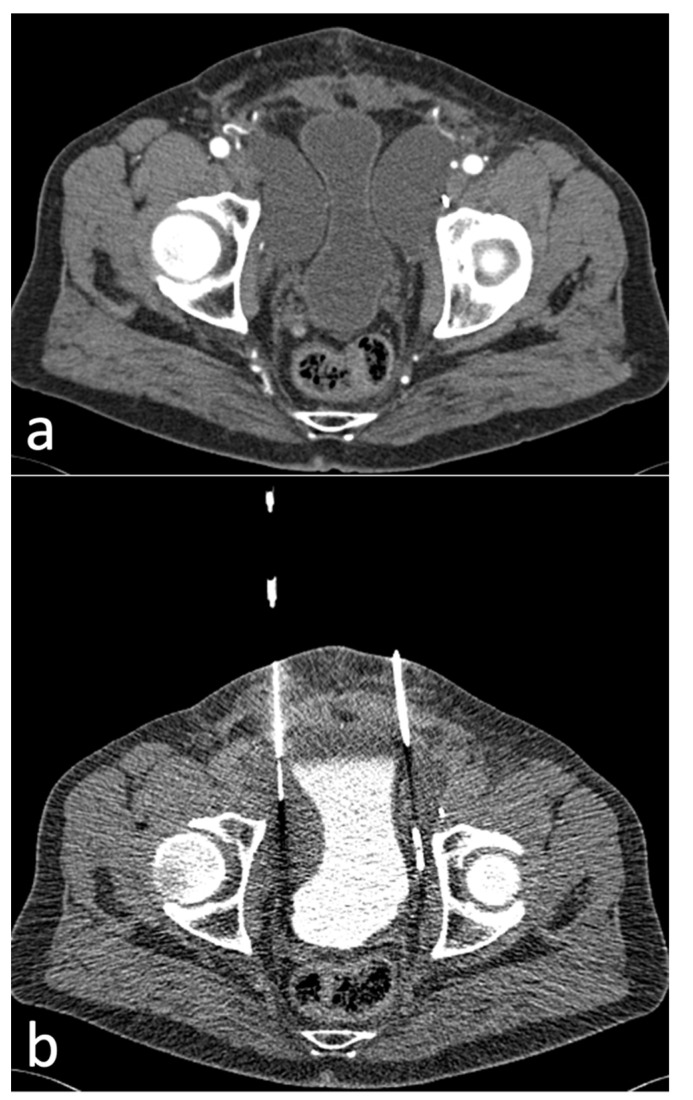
(a)-Axial CT image showing a different patient with bilateral Type 2A lymphoceles compressing the urinary bladder, 20 days after radical prostatectomy with pelvic lymph node dissection. (b)-Axial CT fluoroscopy image showing drainage insertion with the Seldinger technique in the right-sided Type 2A lymphocele using a tube current-time product of 25 mAs. An 8F pigtail drainage lies within the left-sided lymphocele.
